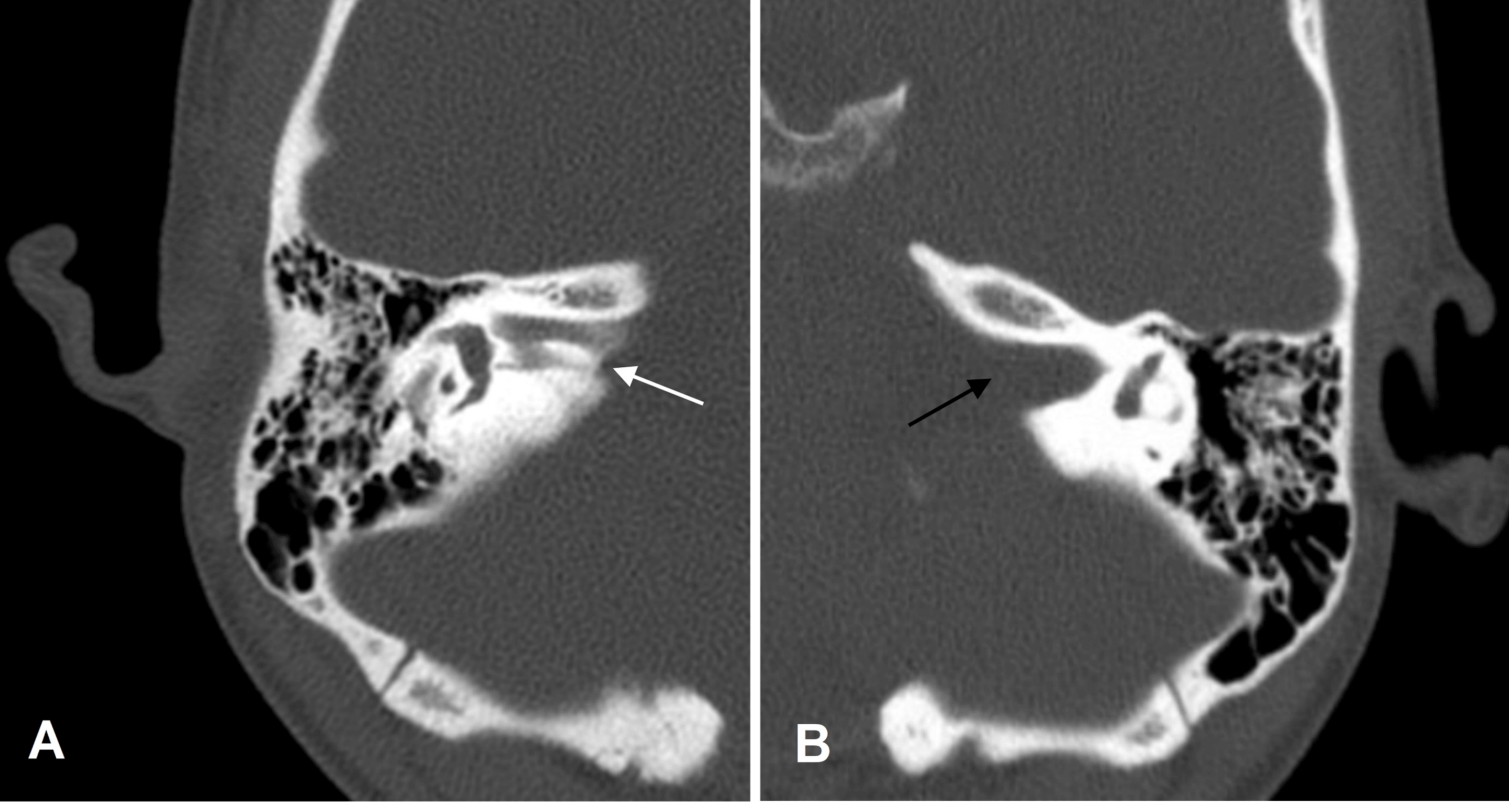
CT temporal bone axial view: the right temporal bone shows (A) a bony prominence (white arrow) within the IAC and (B) a normal, patent IAC on the left side (black arrow) CT: computed tomography; IAC: internal auditory canal
